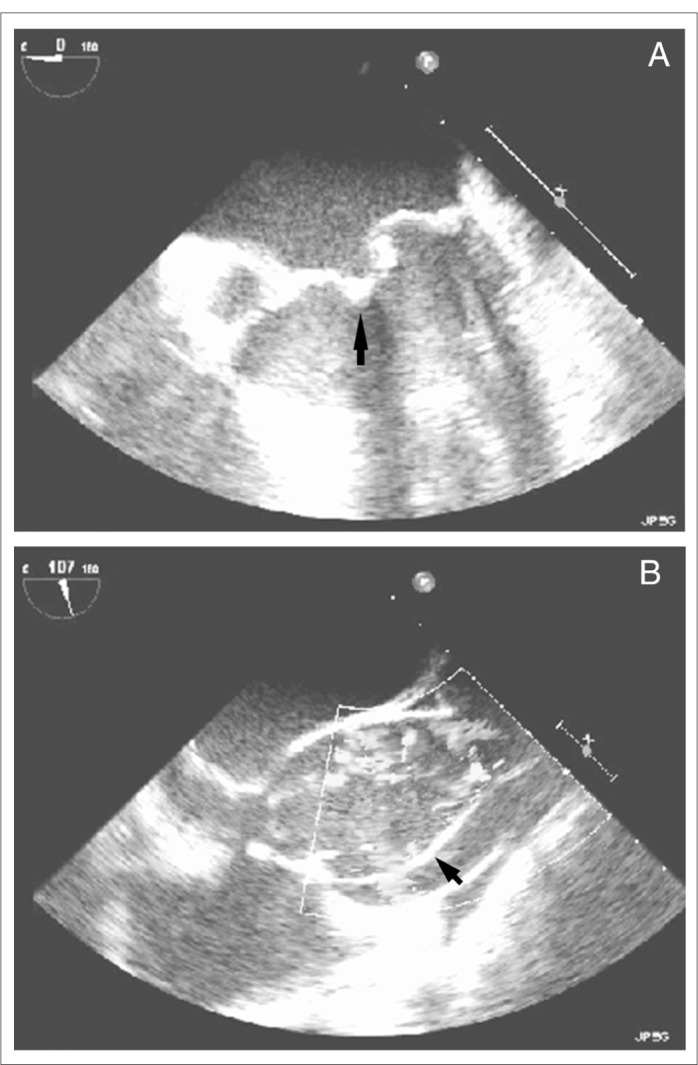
Echocardiography on a 29-year-old male patient with Marfan's syndrome showing (A) mitral valve prolapse (anterior mitral leaflet flail) (arrow) on a four-chamber view, and (B) aortic root dilation and aortic dissection with an intimal flap (arrow) in the aortic cavity on a long-axis view.
